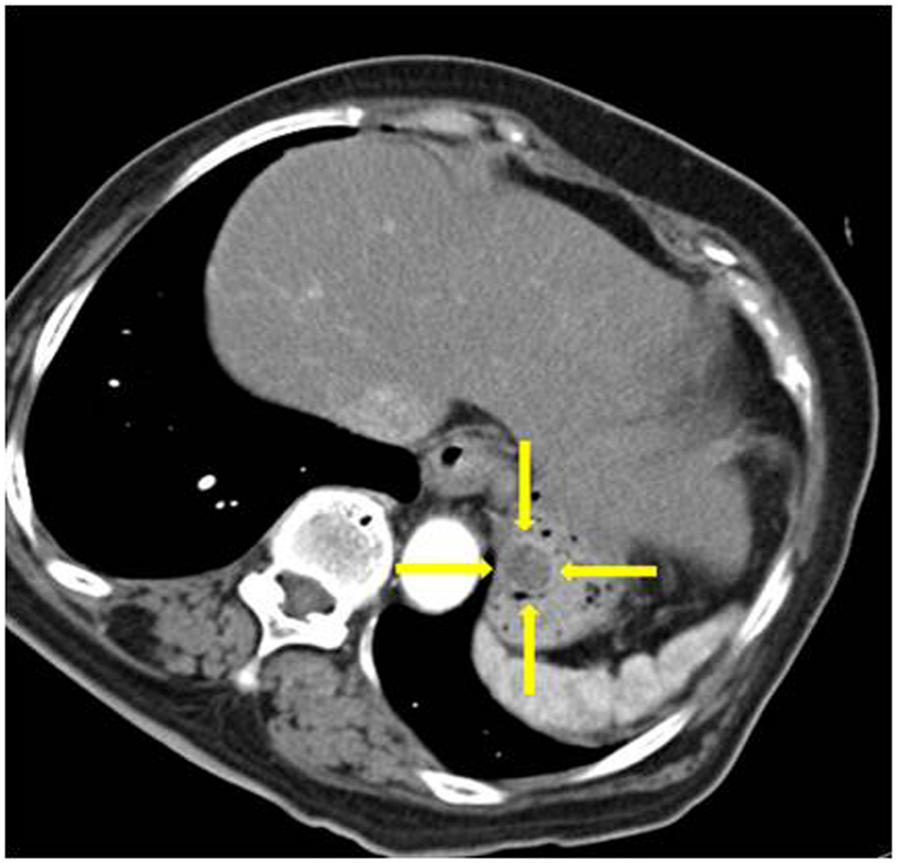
Contrast-enhanced abdominal computed tomographic scan of the patient’s stomach. Image shows a well-defined, heterogeneous, round mass in the stomach body (yellow arrows). Neither regional lymph node enlargement nor distant metastasis can be seen.
